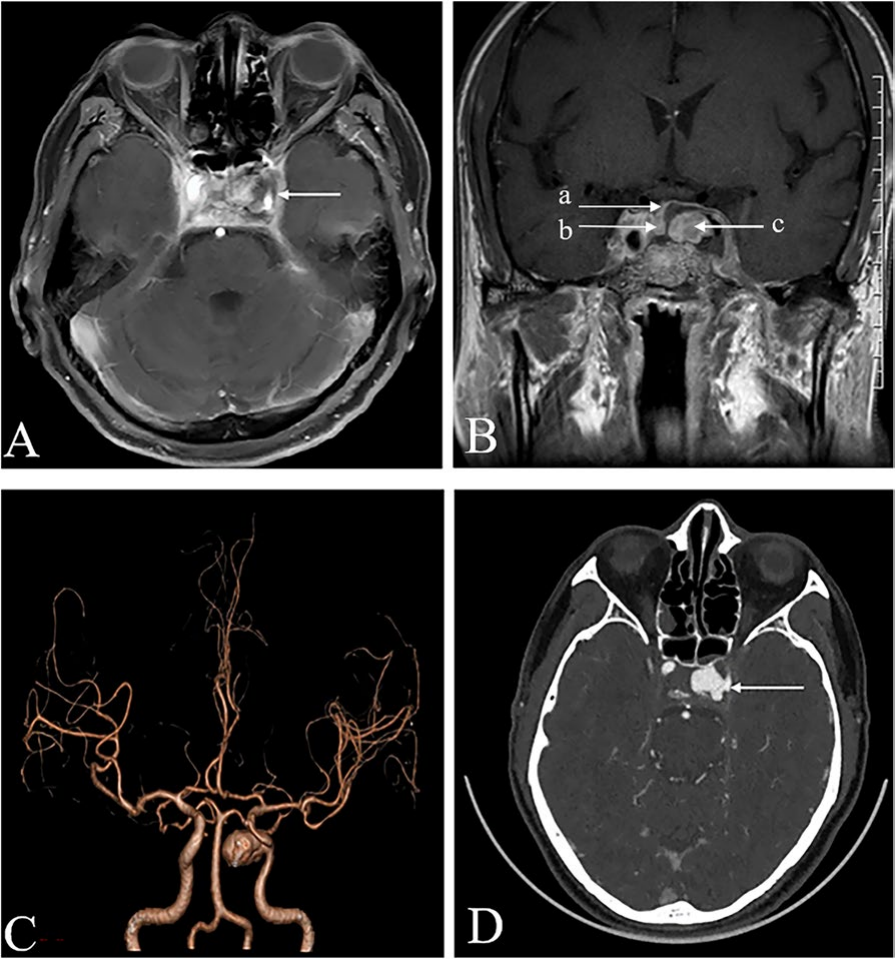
A: an axial contrast-enhanced magnetic resonance image reveals a mass lesion in the left cavernous sinus (arrow). B: Pituitary contrast-enhanced T1-weighted magnetic resonance image shows pituitary compression by the aneurysm (arrow c) and a right shift of pituitary stalk under compression (arrow a). Arrow b shows the pituitary gland. C: Computed tomography angiography shows a large aneurysm in the cavernous sinus segment of the left internal carotid artery about 14.2 × 11.7 mm. D: an axial raw image of computed tomography angiography at the level of biggest diameter of the aneurysm
